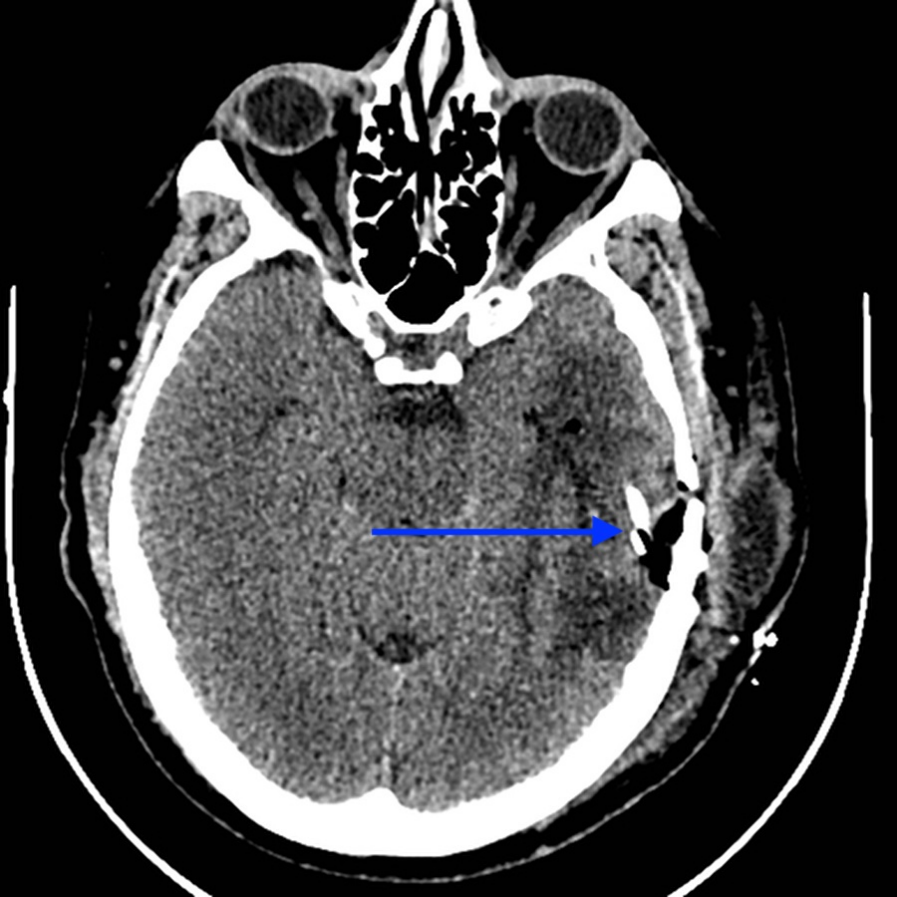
Postoperative unenhanced axial CT of the head: end of shunt tube (blue arrow) in subdural space. CT - computed tomography
